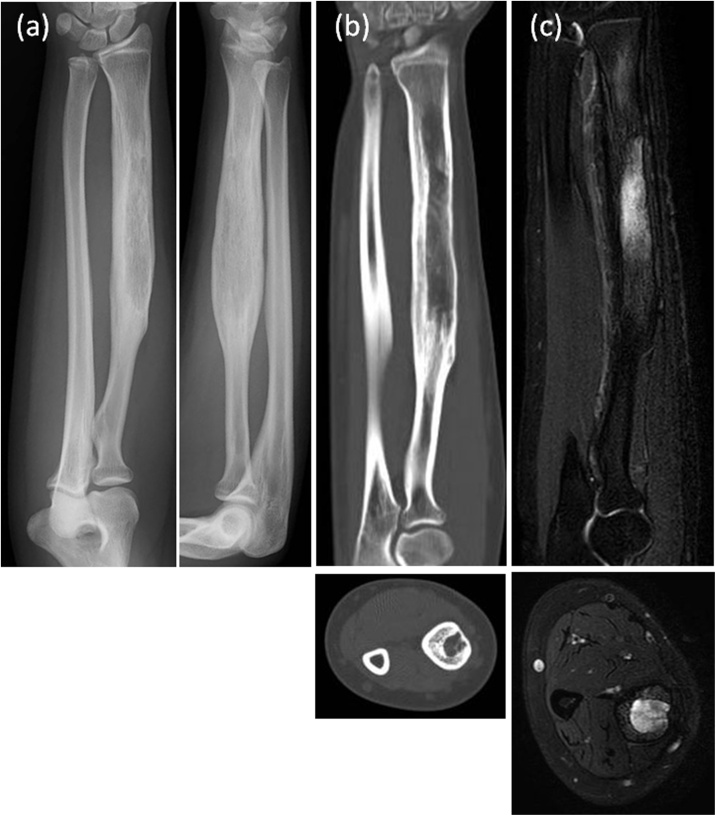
Image findings one year after surgery. (a), (b) Plain radiograph and CT scan show the disappearance of the sclerotic lesion and the recovery of the trabecular structure of the radius. (c) An MRI shows that the extraosseous abscess has disappeared.
